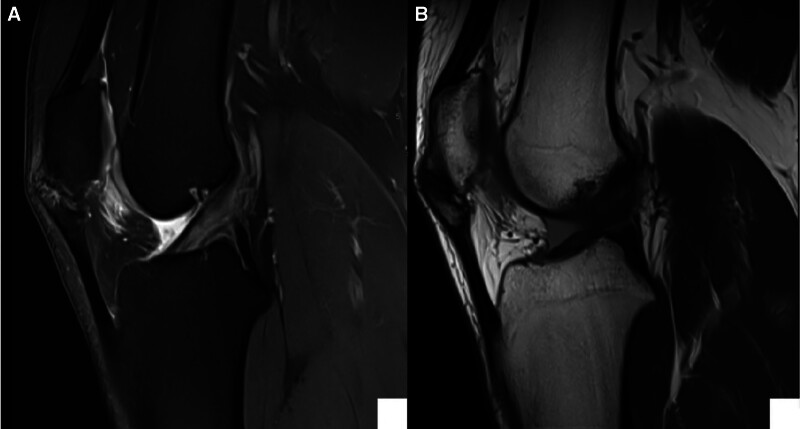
Follow-up MRI of the knee performed 3 years after the initial study, demonstrating progression of the Sinding-Larsen–Johansson lesion at the inferior pole of the patella. (A) Sagittal T2-weighted image showing persistent cortical irregularity with increased fragmentation and surrounding marrow signal changes. (B) Sagittal T1-weighted image highlighting sclerosis and chronic bony remodeling at the patellar tendon insertion site, consistent with long-standing osteochondrosis. MRI = magnetic resonance imaging.
